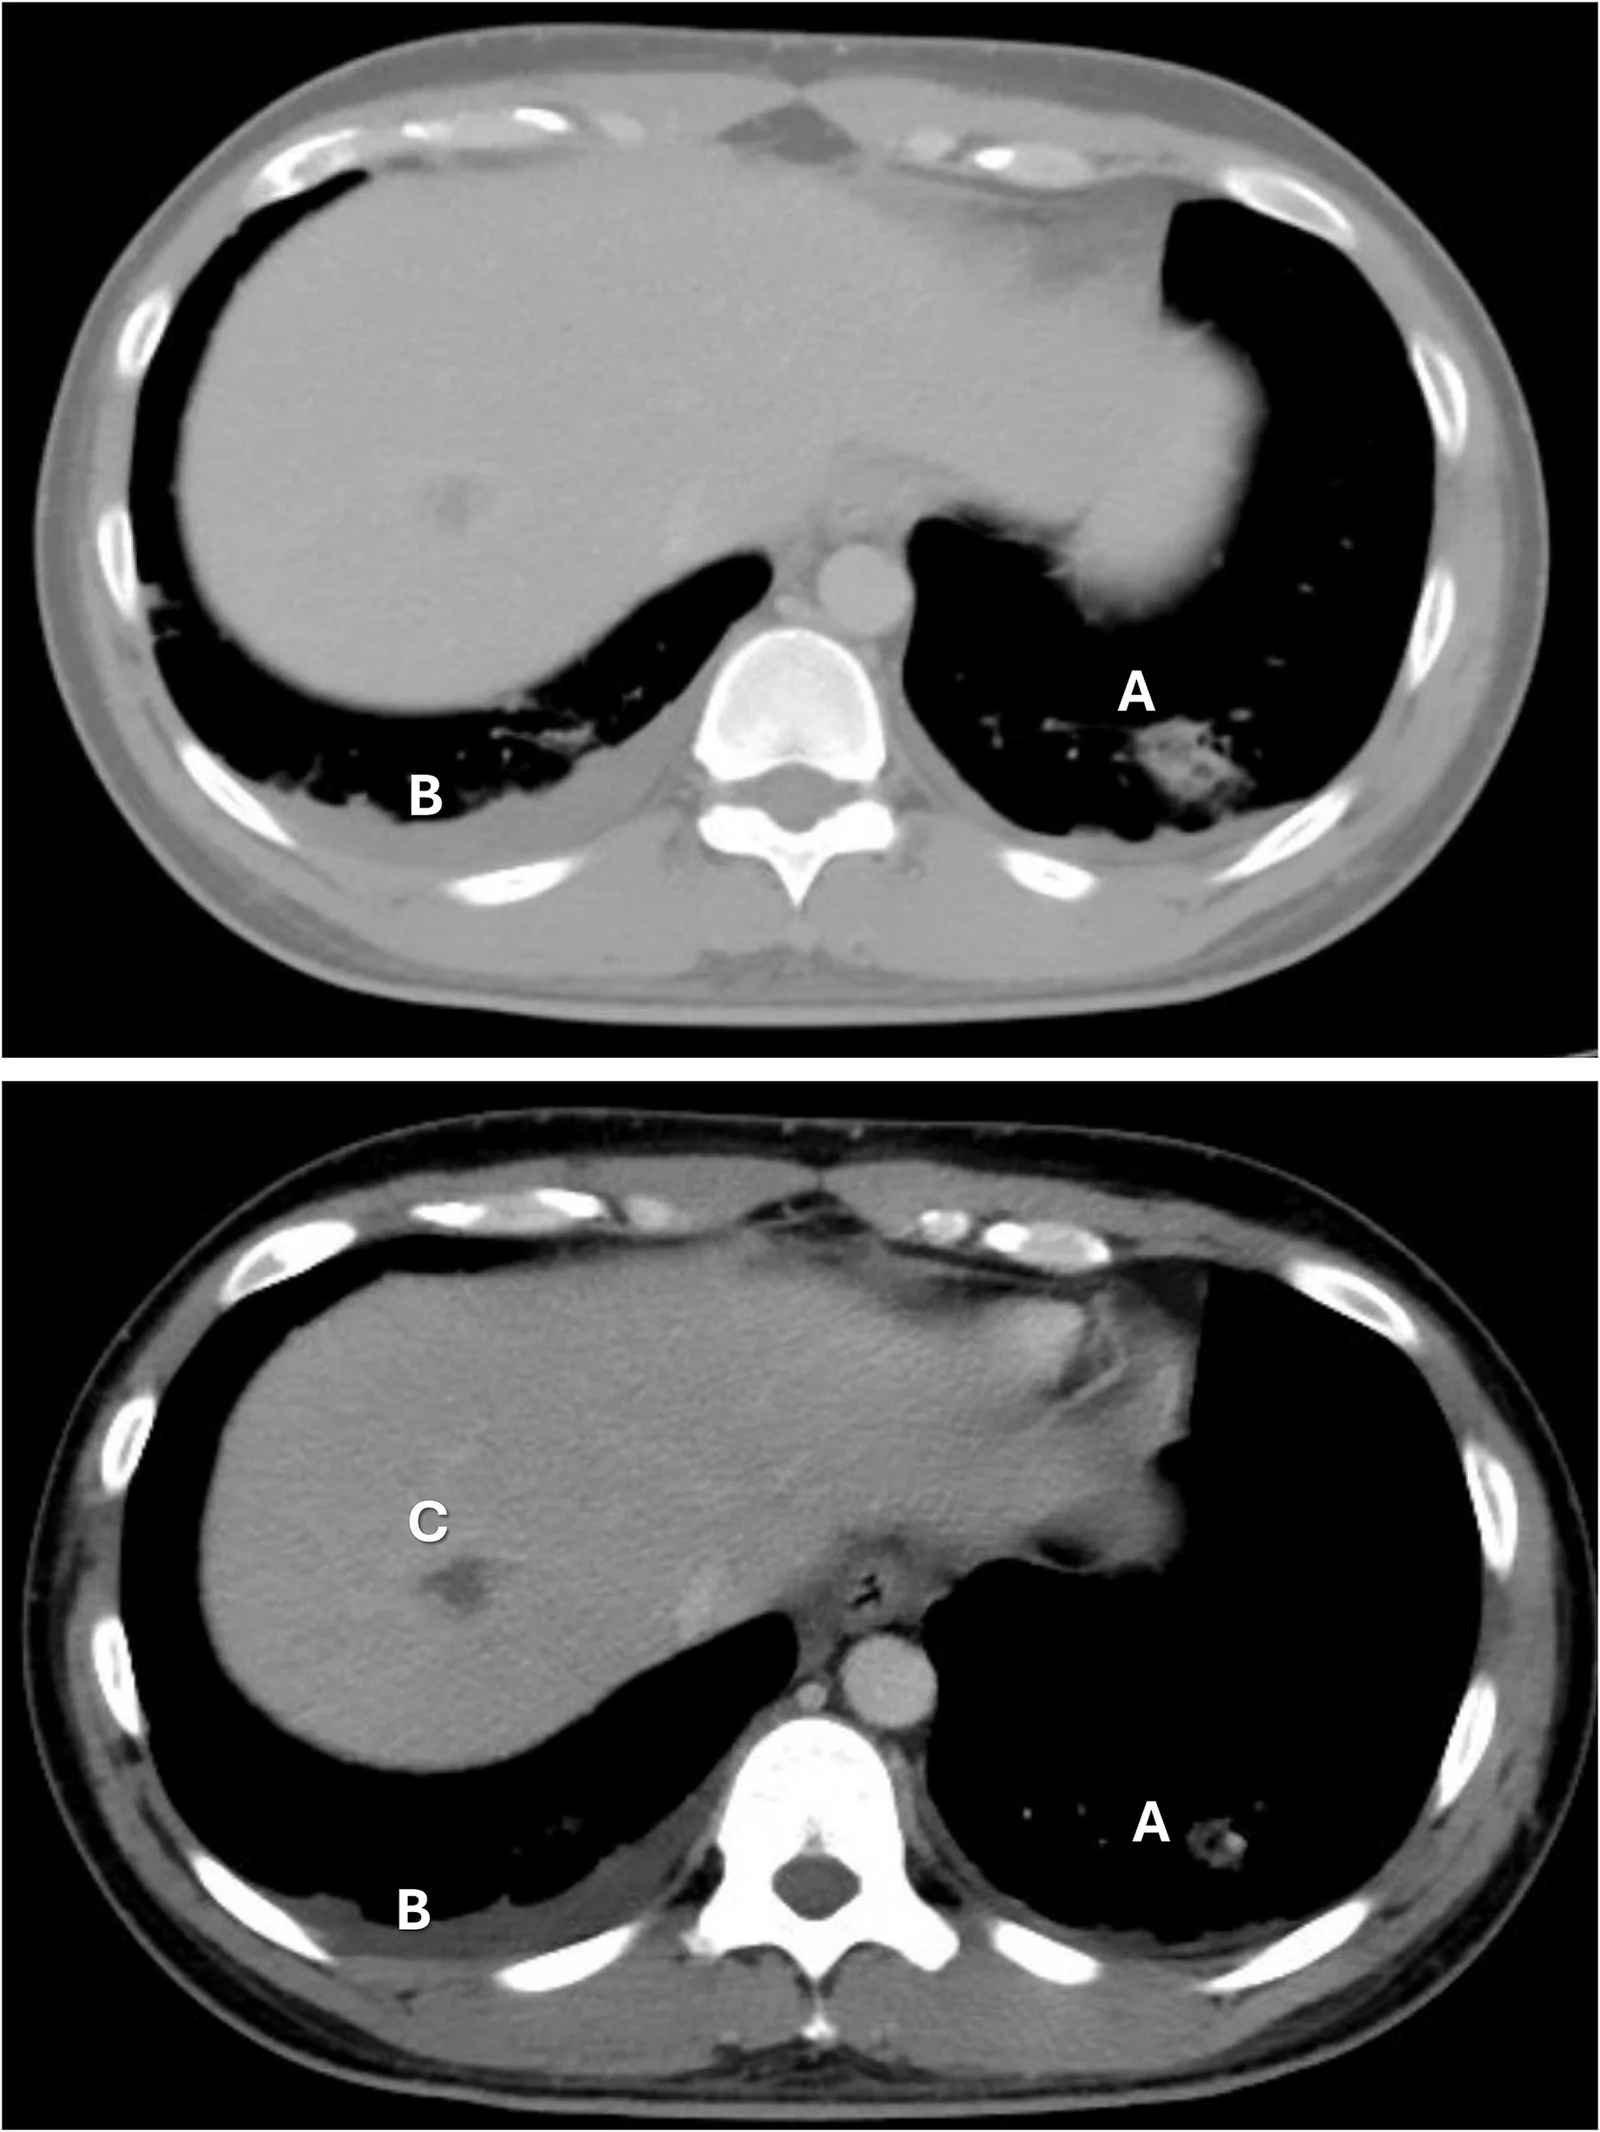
Axial chest and abdominal CT: (A) multiple bilateral peripheral wedge-shaped pulmonary nodules, a pattern characteristic of septic emboli; (B) small right pleural effusion; (C) ring-enhancing hypodense lesion in hepatic segment 8 (S8) dome, consistent with a pyogenic abscess.
